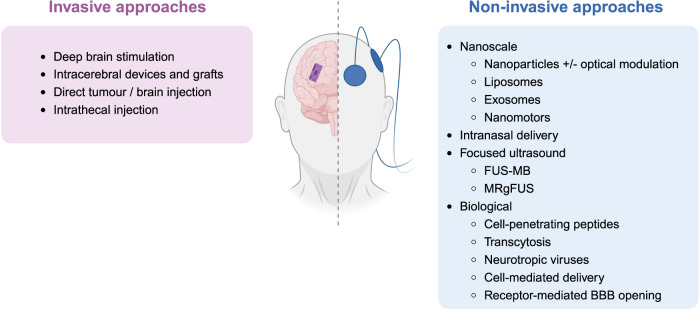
Technologies for delivering therapeutics to the brain, particularly for brain cancer treatment, can be categorised as either invasive or non-invasive. Emerging among these approaches are nanoscale technologies that serve as experimental tools for circumventing the BBB to facilitate drug delivery into glioblastoma. FUS-MB focused ultrasound, with intravenous administration of microbubbles; MRgFUS MRI-guided focused ultrasound^5^.
